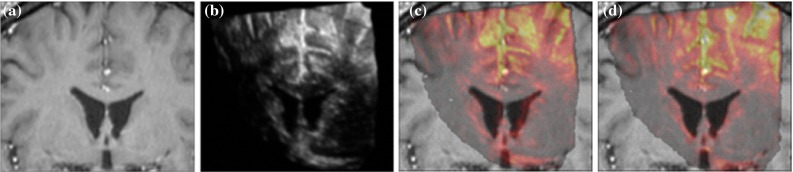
Deformable MRI-US registration results of case 1 using miLBP. a MRI slice. b The corresponding US slice before registration. The corresponding US slices before and after deformable registration shown in pseudo-color are overlaid on the MRI image in c and d, respectively
